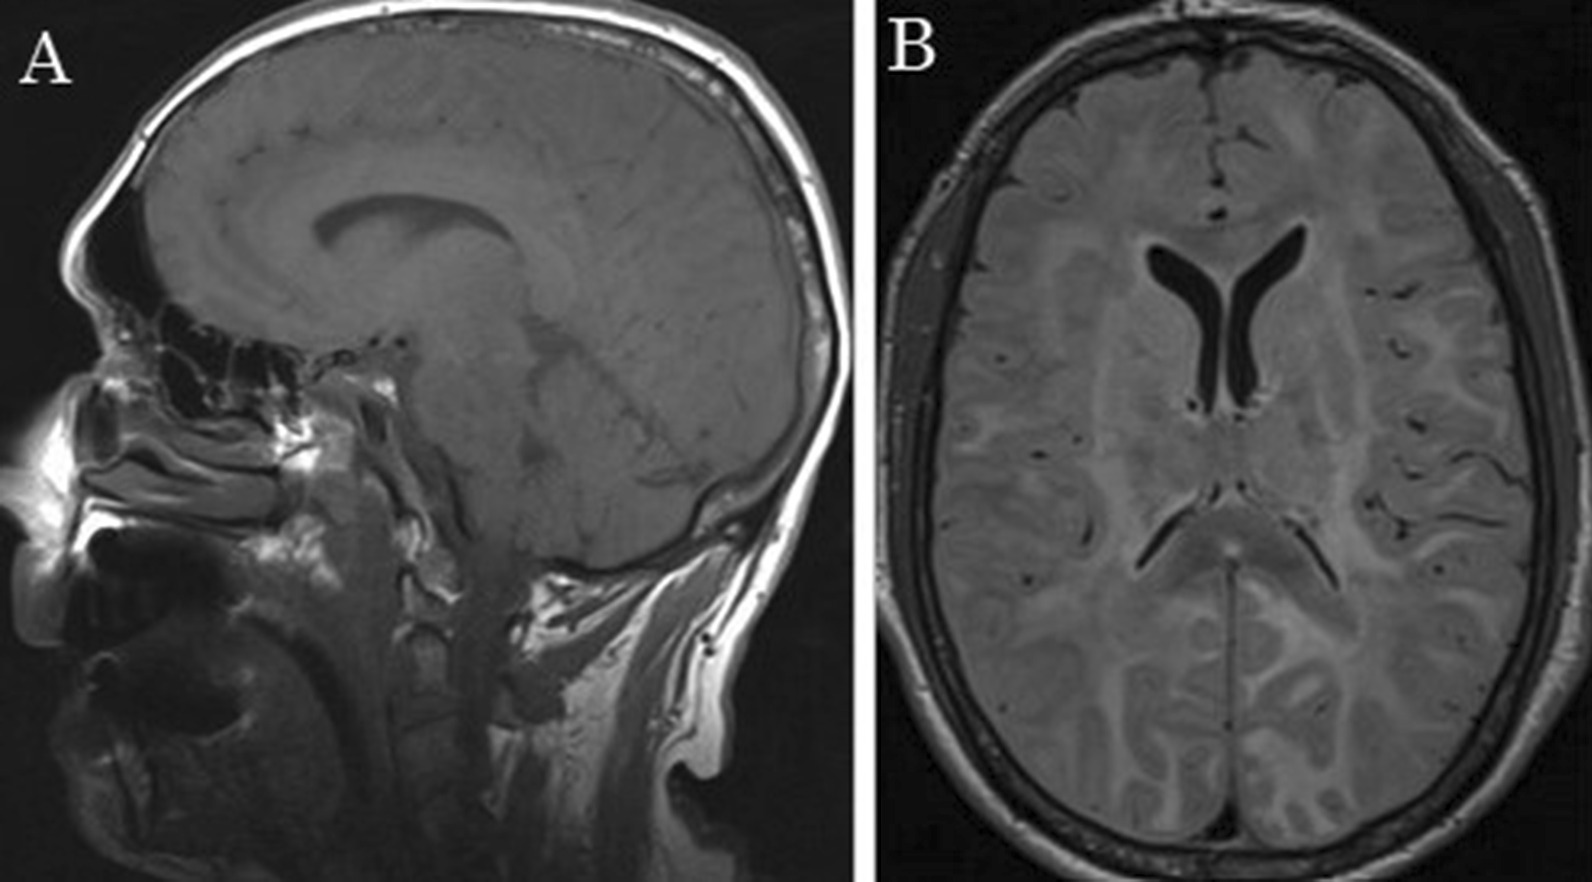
MRI T1 and T2 sequences with extensive involvement of supra- and infratentorial white matter, with bilateral and asymmetric damage, deep and superficial, of corpus callosum and internal capsules. A sagittal plane. B axial plane
